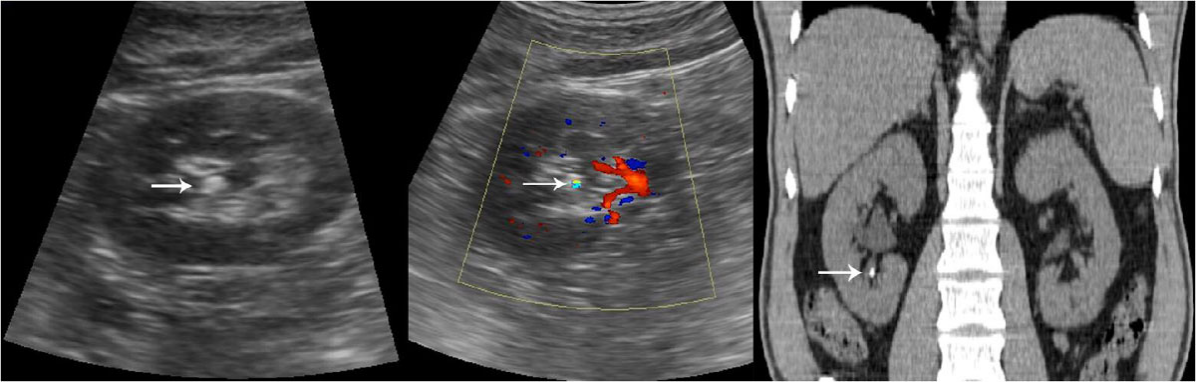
Gray-scale sonogram shows hyperechoic spot with posterior acoustic shadowing. Color Doppler sonogram shows a twinkling sign. A small renal stone. Unenhanced CT confirms the presence of renal stone.
